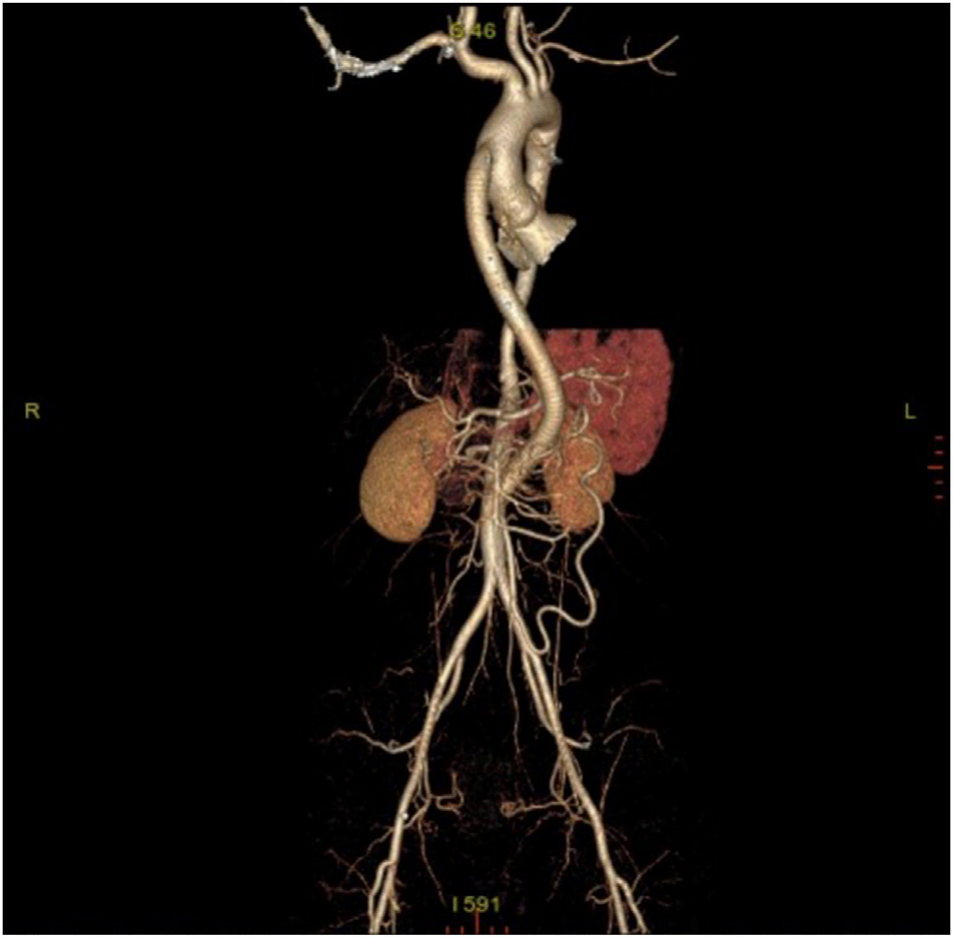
Post-operative computerized tomographic angiography (CTA) of patient with TA.
